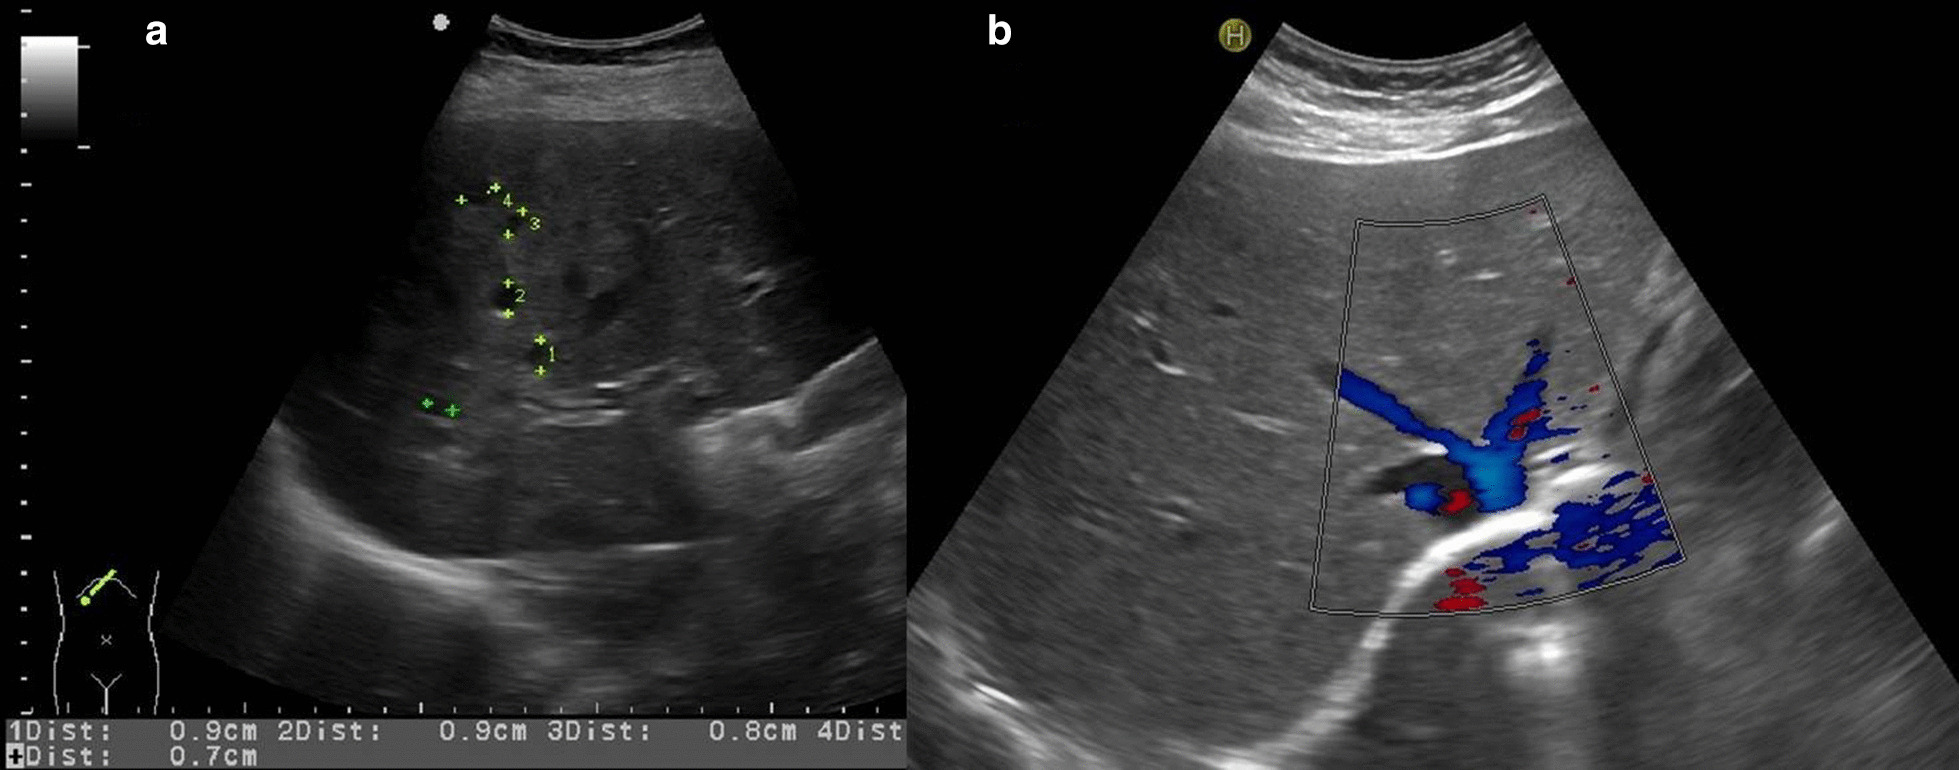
a B-mode ultrasound with hypoechoic liver lesions in the right liver lobe. b Normal liver tissue after successful antibiotic therapy
